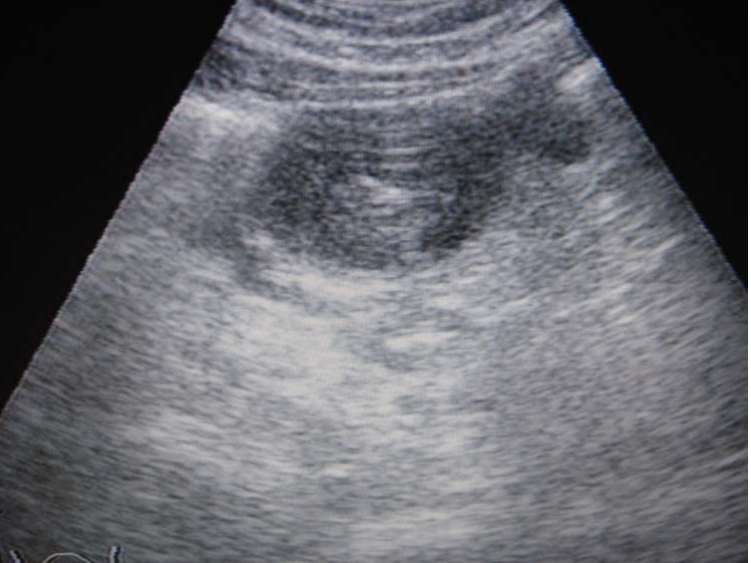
Preoperative ultrasound showing the large pseudopolyp in the jejunoileal region.
